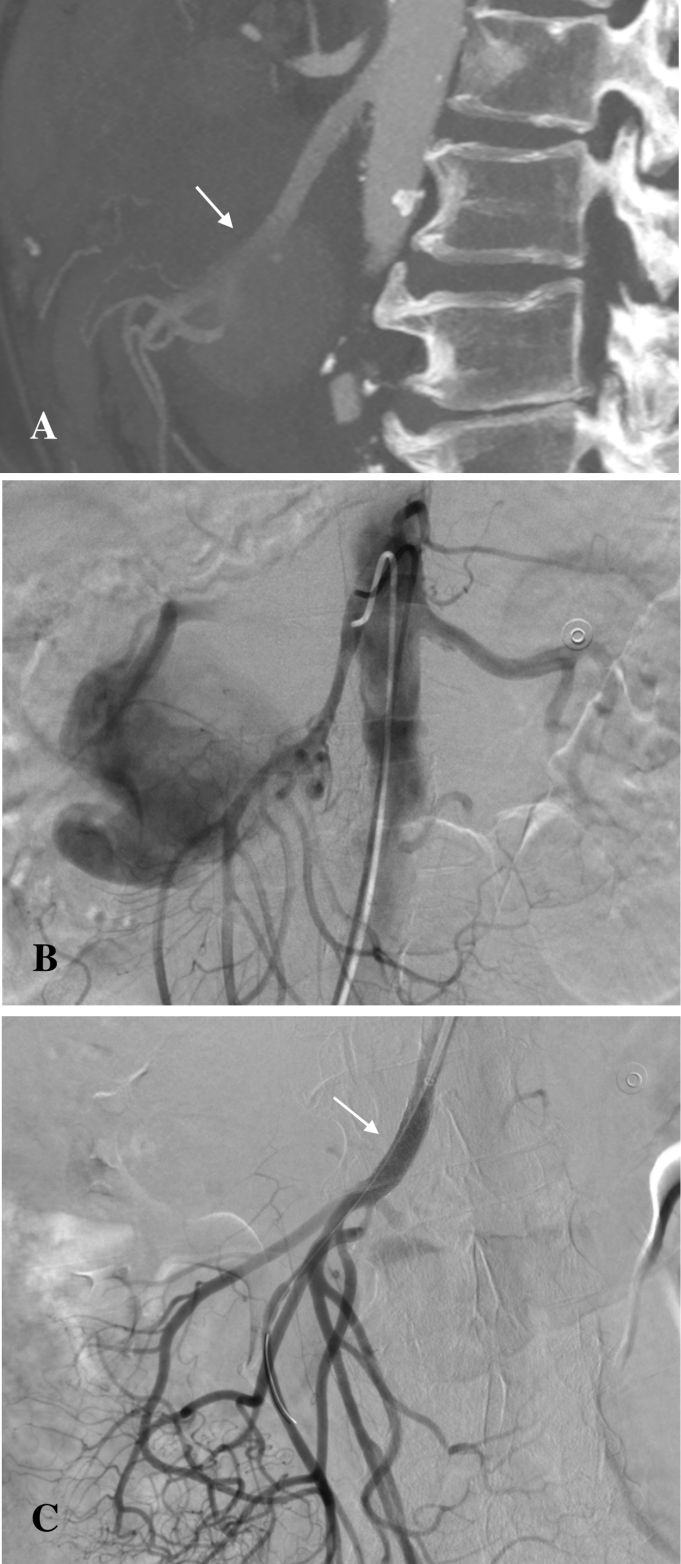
Superior mesenteric artery pseudoaneurysm treated with stent graft implantation. (A) Contrast-enhanced CT scan showing the pseudoaneurysm arising from the SMA (arrow). (B) DSA showing pseudoaneurysm. (C) Final selective DSA confirming the exclusion of the pseudoaneurysm following stent graft implantation and post-dilation.
